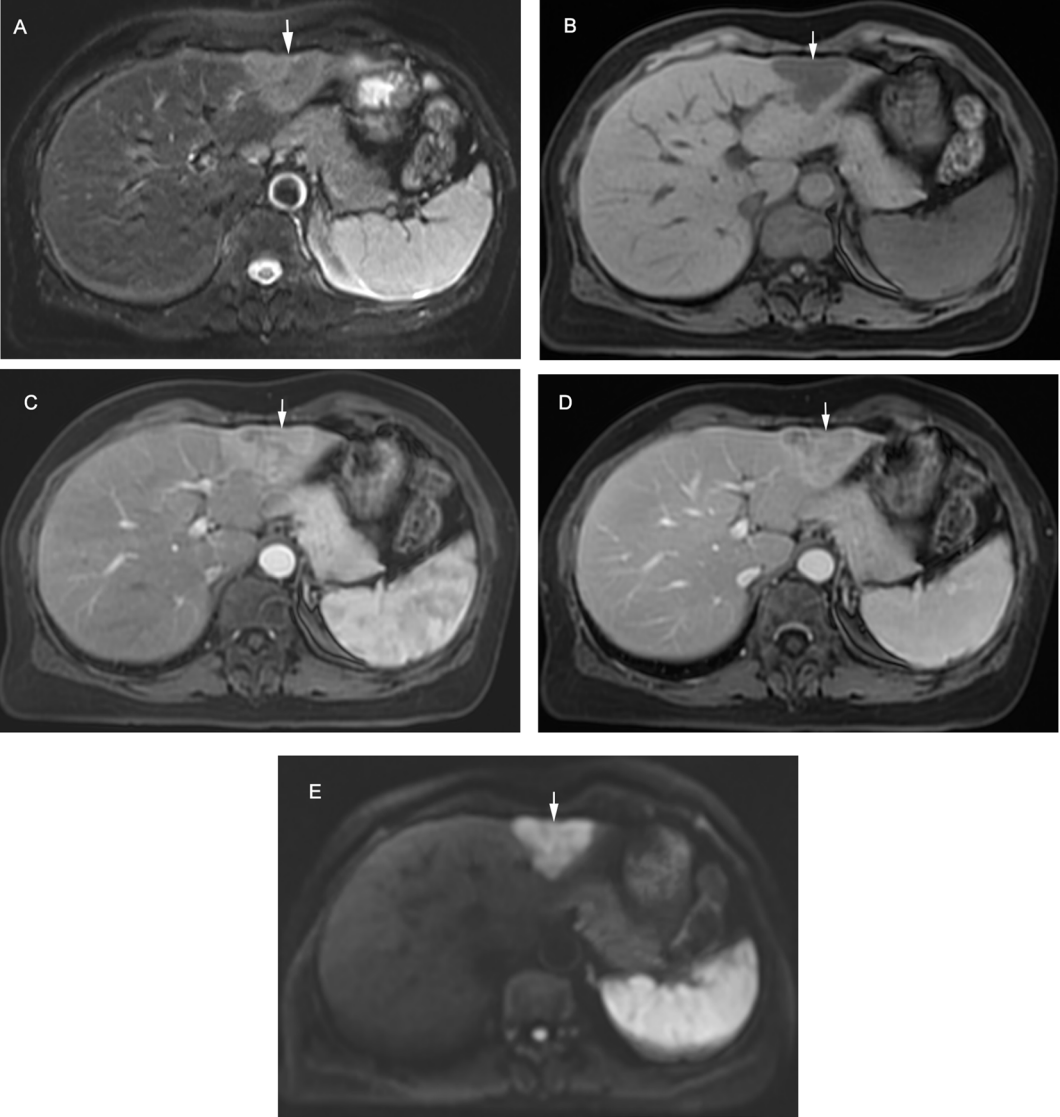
Intrahepatic cholangiocarcinoma in a 59-year-old woman (white arrows). (A) T2-weighted image shows a mild hyperintense tumor. (B) T1-weighted image shows a hypointense tumor. Contrast-enhanced (C) arterial phase image shows non-rim hyperenhancement; (D) delayed image shows a washout enhancement pattern with enhancing capsule. (E) Diffusion-weighted image (b = 500 s/mm^2^) shows non-targetoid restriction. The lesion is categorized as LR-5
